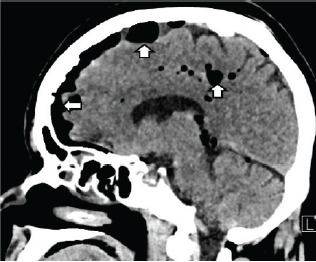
Sagittal image from the patient’s non-contrast computed tomography of the head depicting intracranial air (white arrows).
